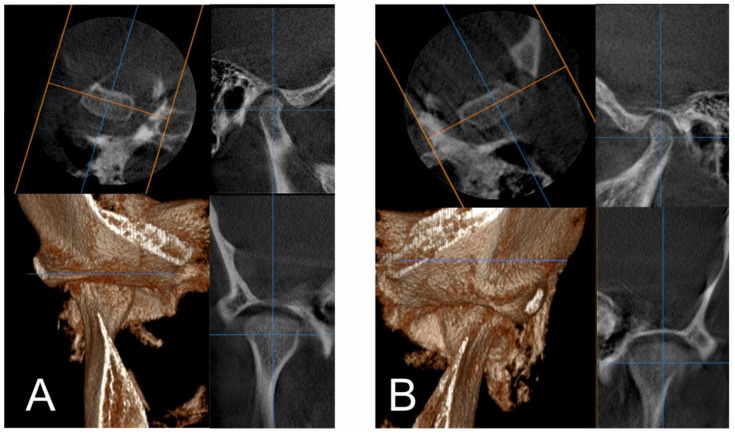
Pre-treatment TMJ aspect on CBCT: right (A) and left (B) TMJ with coronal, sagittal, axial cross-sections and 3d reconstruction.
